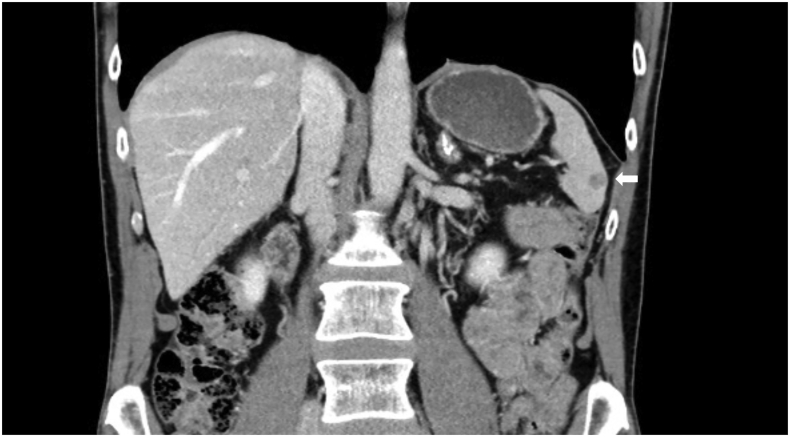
CT-image showing the splenic metastasis to be removed (white arrow).
